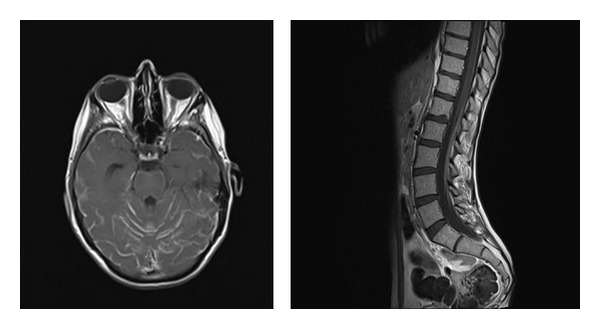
Postcontrast T1-weighted MRI images of diffuse enhancement in cerebral sulci and linear enhancement surrounding the dorsolumbar spinal cord and the lumbosacral roots in a 28-yr-old female with breast cancer.
